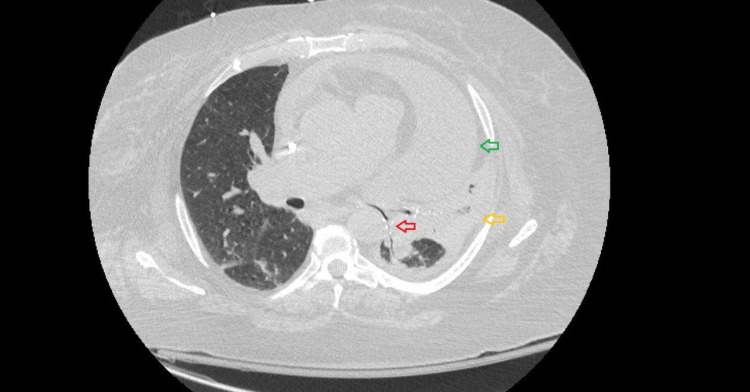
Computed tomography of thorax with a small left-sided pleural effusion (yellow arrow) and a large pericardial effusion (green arrow) compressing on the left bronchus and adjacent lung (red arrow)
